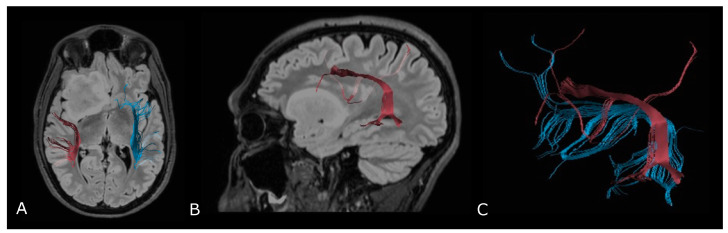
Tractographic reconstruction of arcuate fasciculi. DTI of the direct pathway of both arcuate fasciculi (AF) fused with anatomic axial (A); and sagittal (B) FLAIR images. The right AF on the affected side is superiorly and posteriorly displaced by an IDH-mut frontal-temporal-insular astrocytoma; (C) 3D rendering of both arcuate fasciculi.
